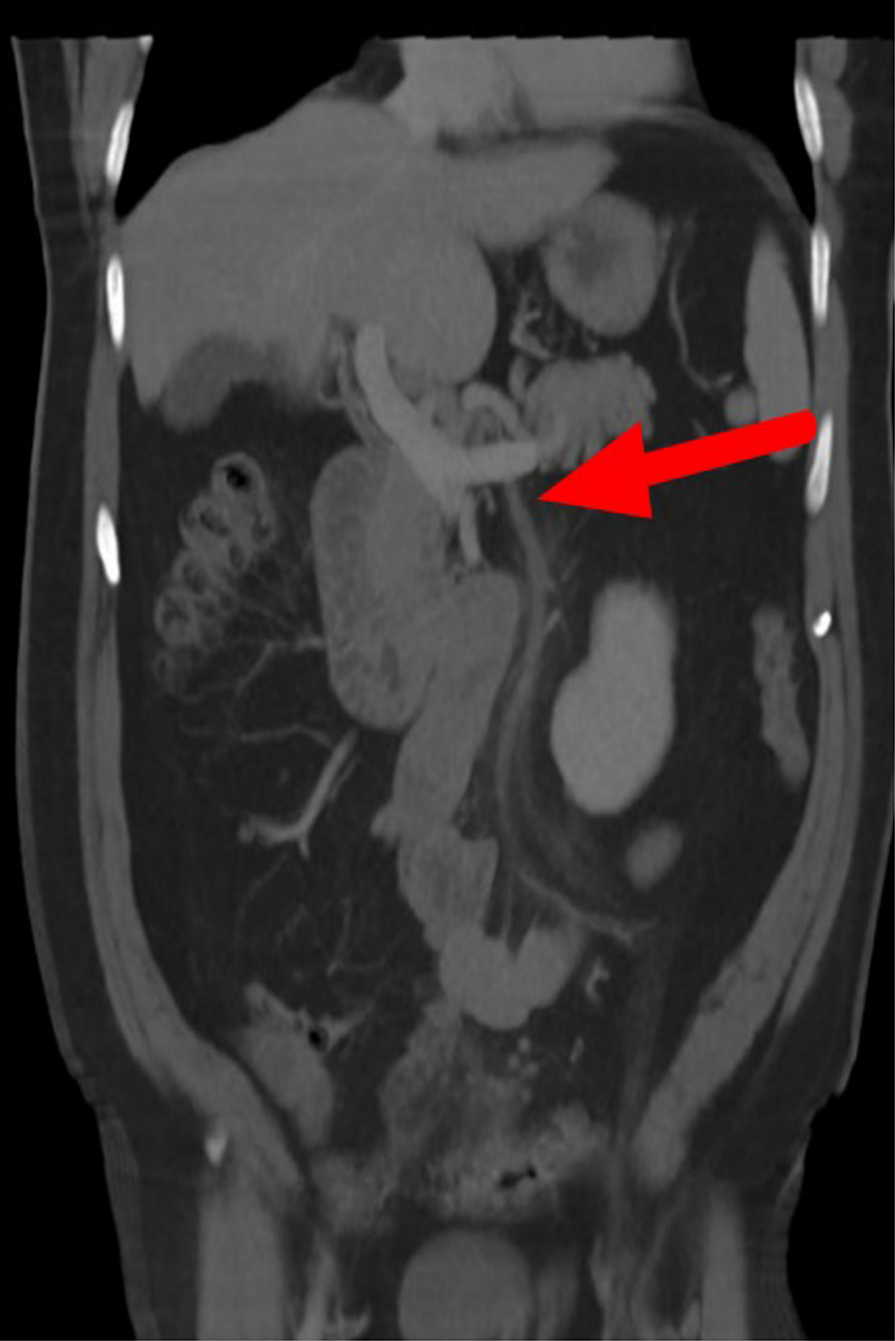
CT abdomen/pelvis 3D coronal view demonstrating acute/early subacute partially occlusive thrombosis of the inferior mesenteric vein with mild adjacent perivascular stranding.
